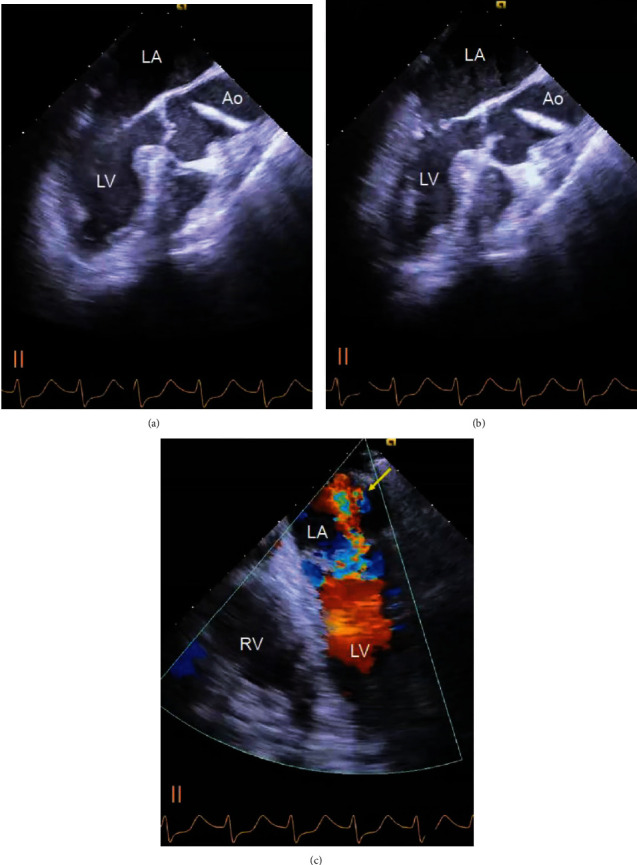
Transesophageal echocardiography. (a, b) Severe hypokinesis of the anteroseptal wall was observed at a 130° angle in a (diastole) and b (systole). Left ventricular ejection fraction was measured as 20%. LA: left atrium; LV: left ventricle; Ao: aorta; RV: right ventricle. (c) Severe mitral regurgitation at a 0° angle (arrow).
